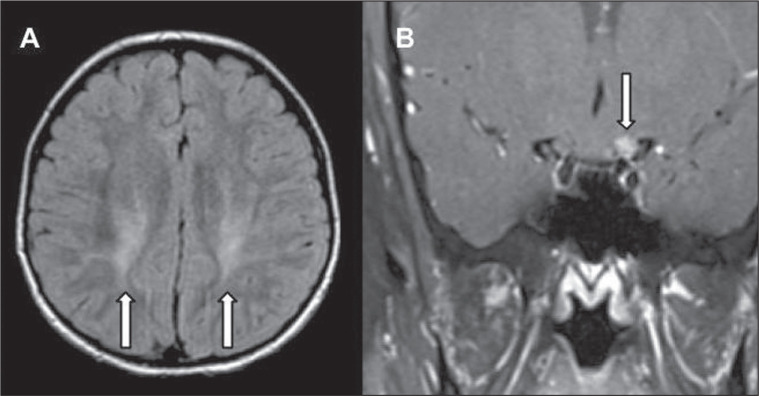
Cranial nerve involvement in INMDs. MRI scan. Axial T2-weighted FLAIR
